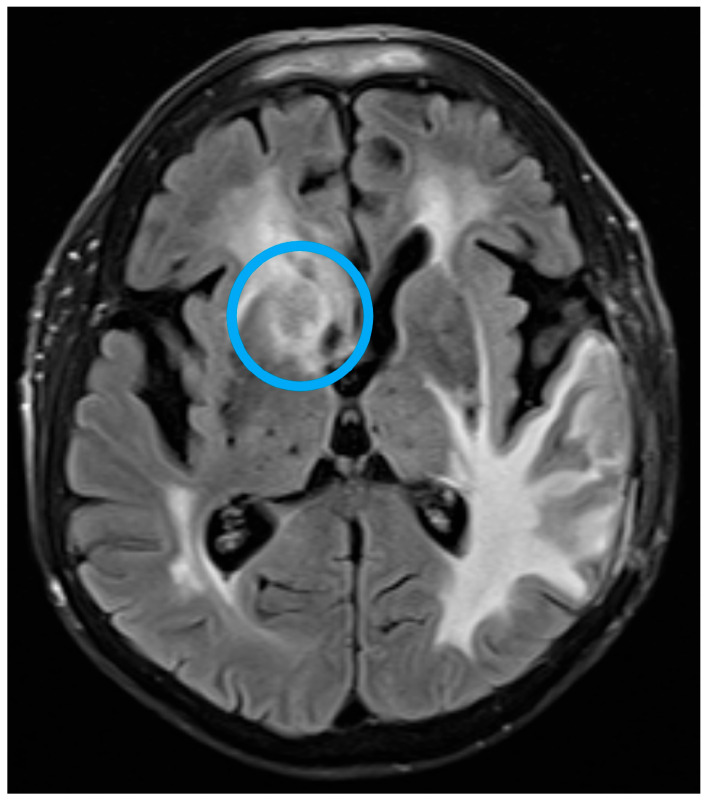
Post-surgical brain MRI—progressive inflammatory lesions consistent with cerebral toxoplasmosis.
